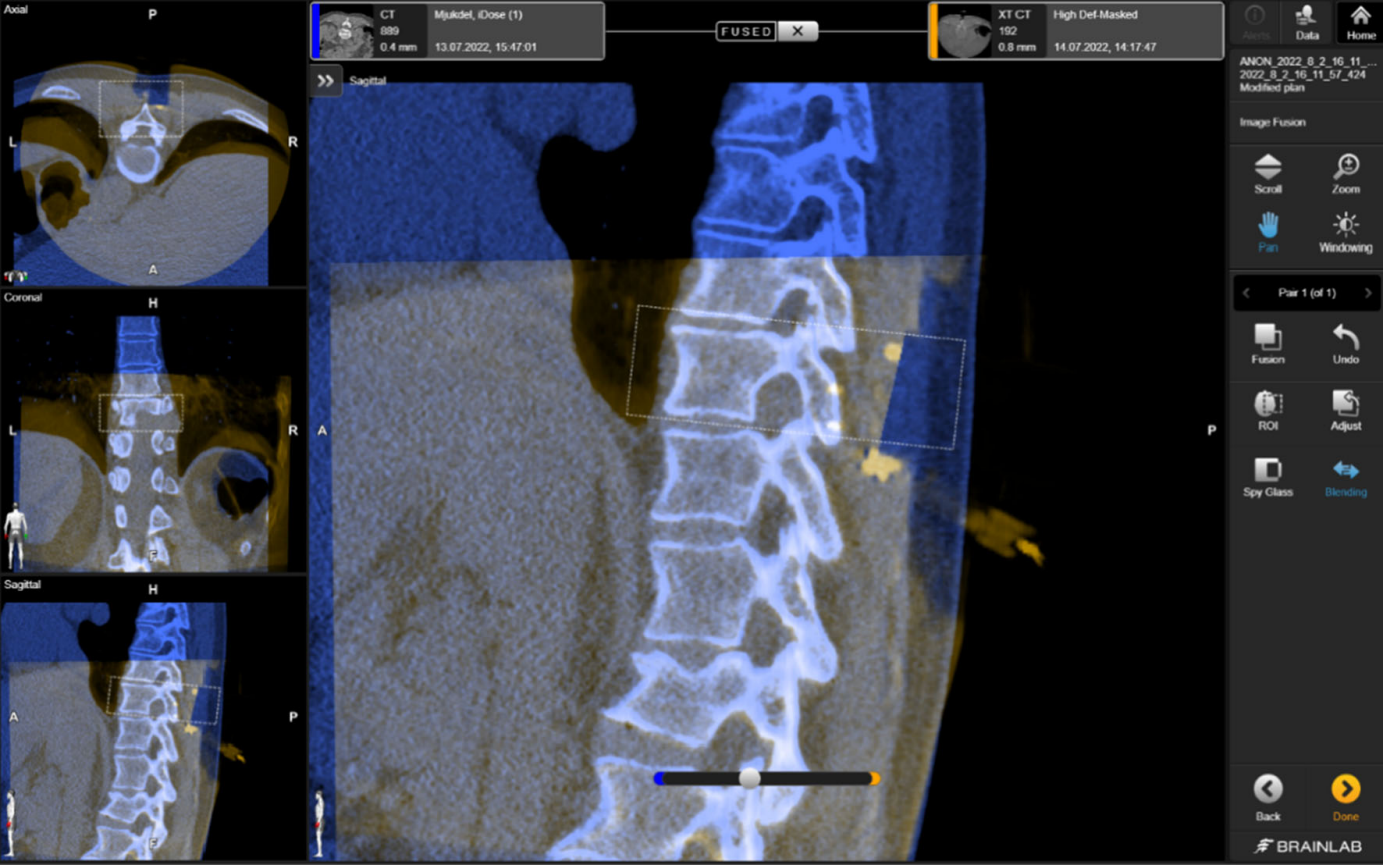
Image fusion of the vertebra of interest between the preoperative CT scan (blue) and the intraoperative CBCT scan (orange). Please note the visible bone screws on the lamina in the region of interest (orange)
